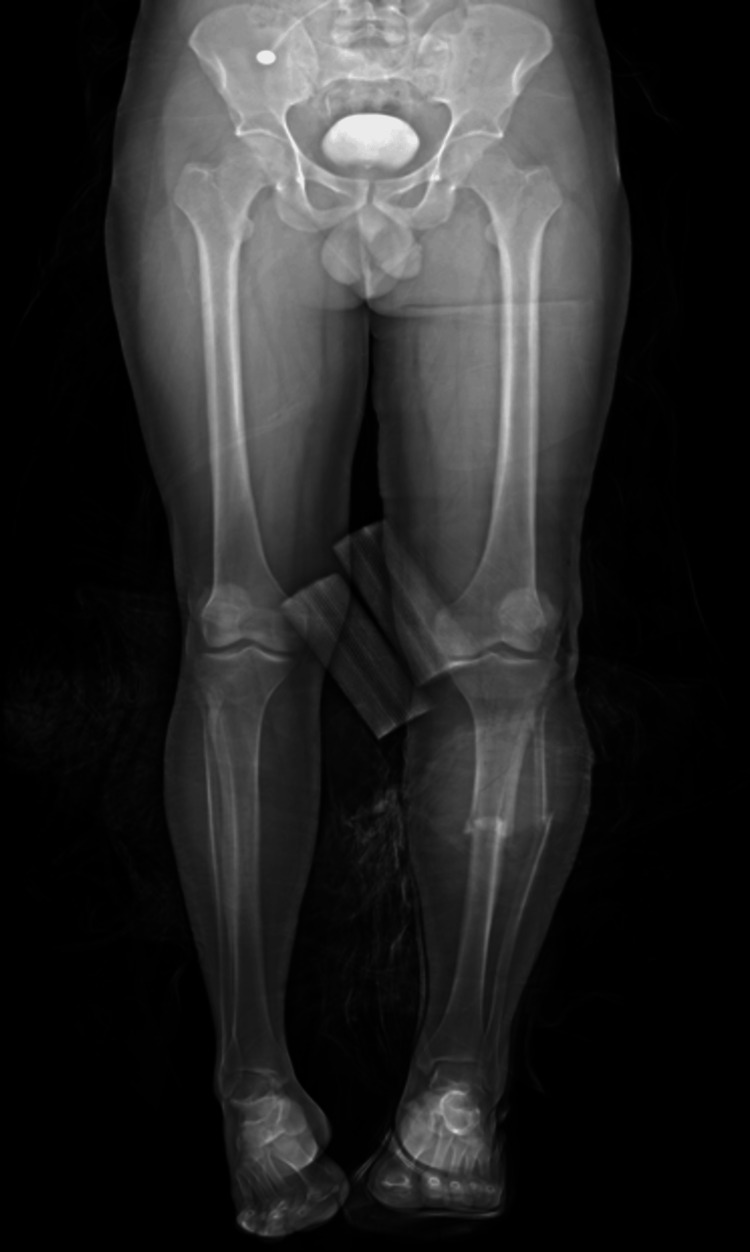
X-ray of the lower limb revealed a fracture of the left tibia.
